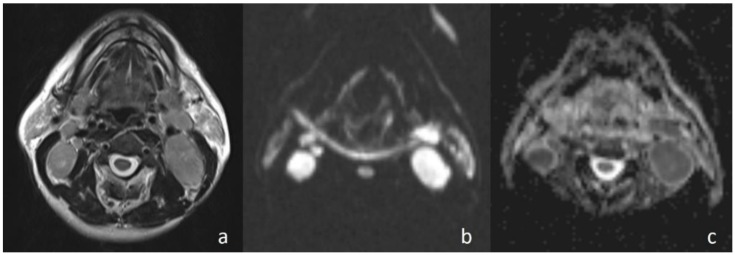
Undifferentiated nasopharyngeal carcinoma. (a) Axial T2WI shows bilateral metastatic cervical lymph nodes; (b) lymph node, on the left side of the neck, shows high signal intensity on DWI; and (c) the mass is hypointense on the ADC map (ADC value of 0.91 × 10^−3^ mm^2^/s).
